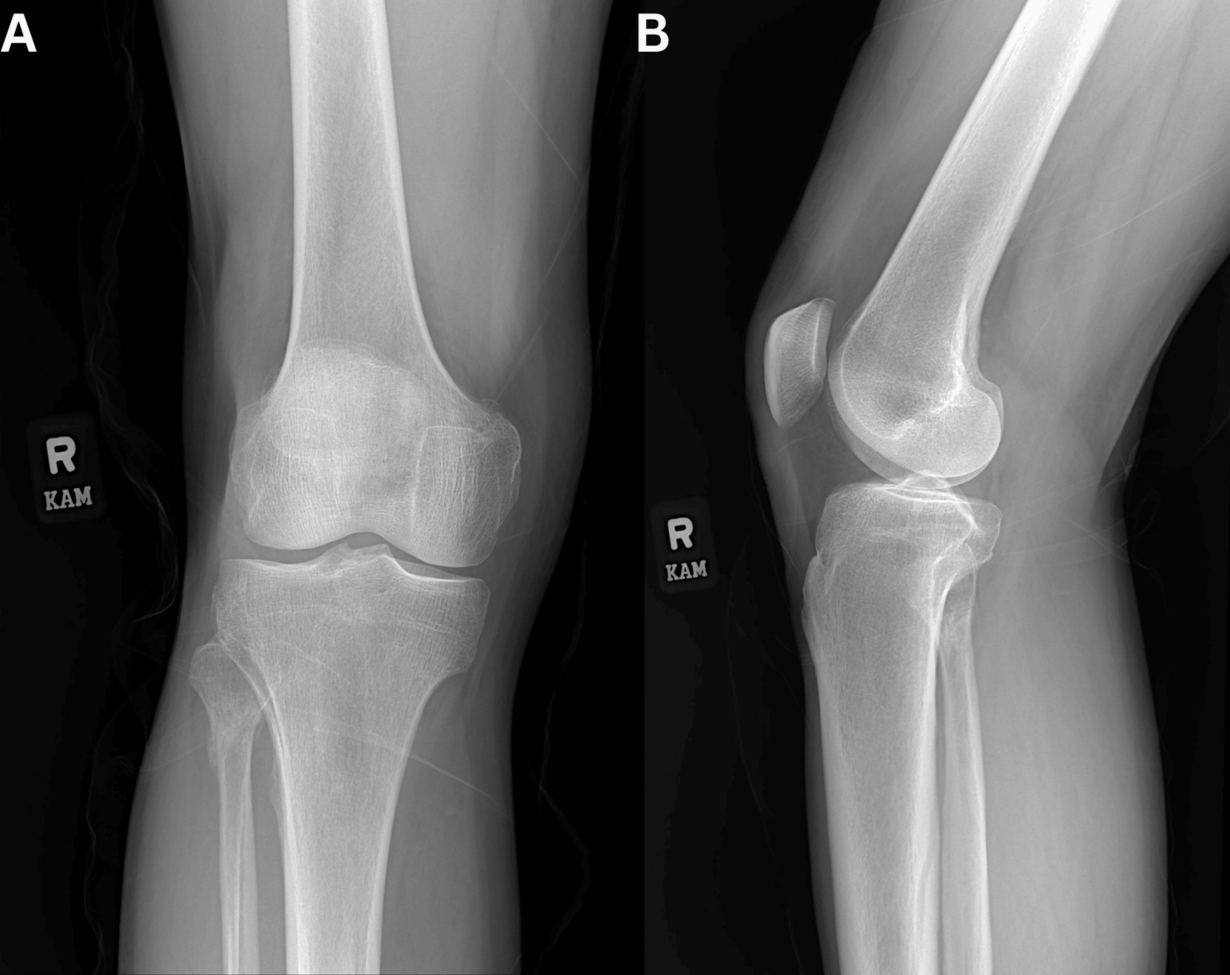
Final follow-up X-ray of the right knee. (A) Anteroposterior and (B) lateral X-ray views of the right knee, taken 10 weeks after the injury, showing bridging callus formation across the proximal fibular fracture. The healing fracture is in normal alignment.
